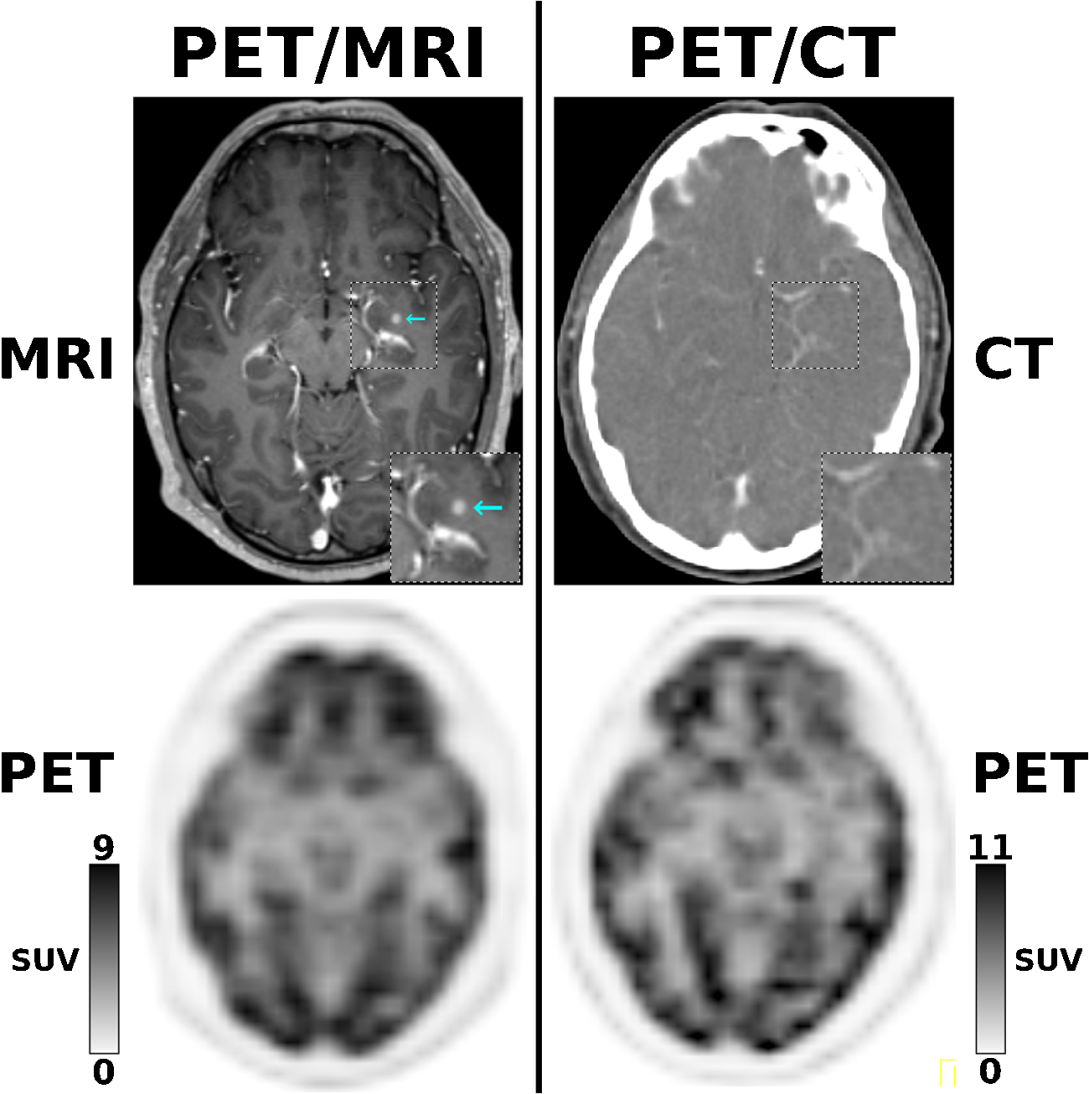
A 61-year-old patient with NSCLC stage IV, referred for staging before nivolumab treatment. While [^18^F]FDG-PET is unremarkable for both PET/MRI and PET/CT, the contrast-enhanced MRI component of PET/MRI depicts a small brain metastases in the left hippocampus (cyan arrow) that is not visualized on the contrast-enhanced CT component of PET/CT (× 1.5 magnifications in right lower corners), and for which radiation therapy is indicated
